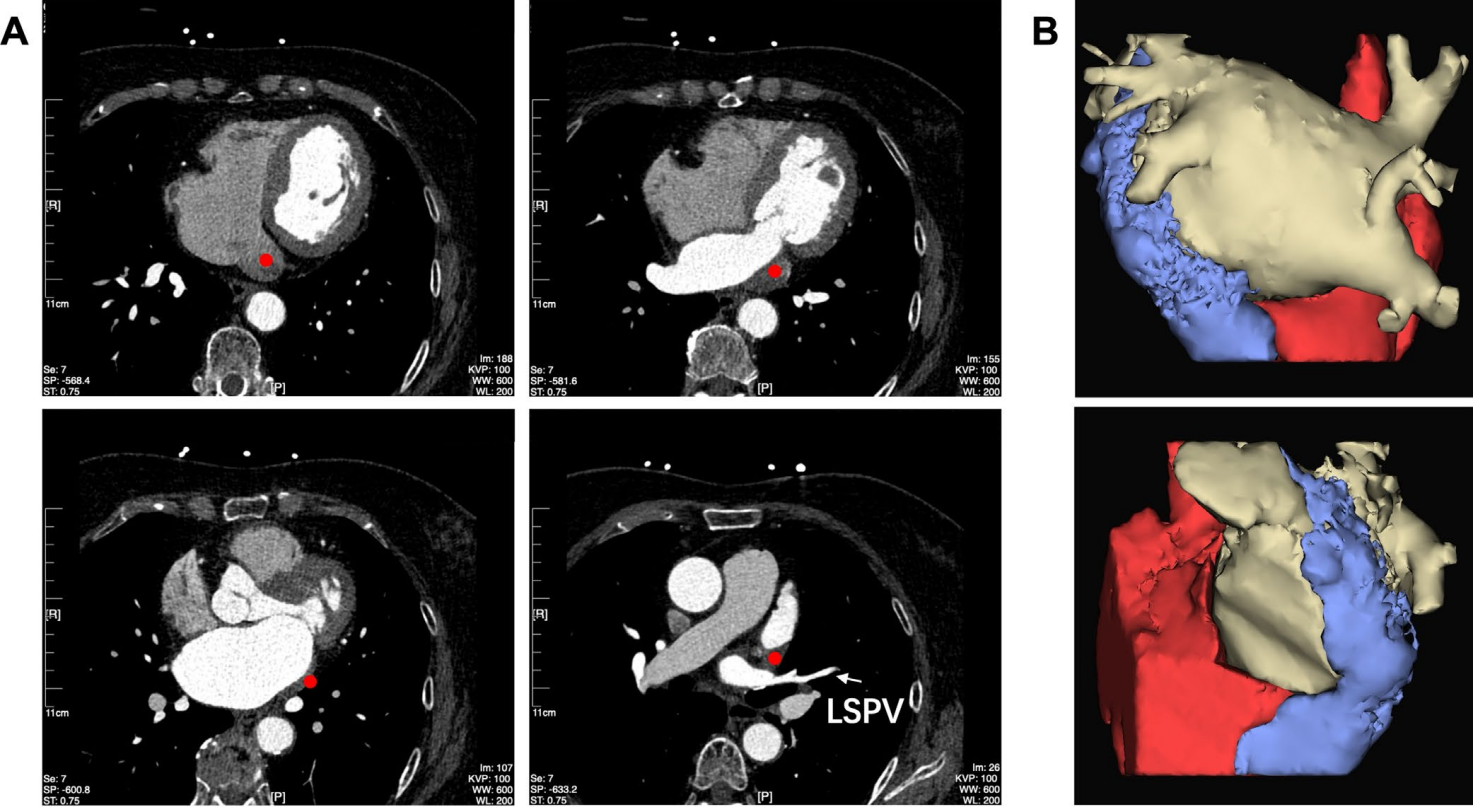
Preprocedural imaging confirmed the presence of PLSVC. (A) Coronary computed tomography angiography (CCTA) slice images, with red dots on each slice indicating the course of the PLSVC. (B) Three‐dimensional CCTA reconstruction showing the left atrium (white), right atrium and coronary sinus ostium (red), and PLSVC (blue).
